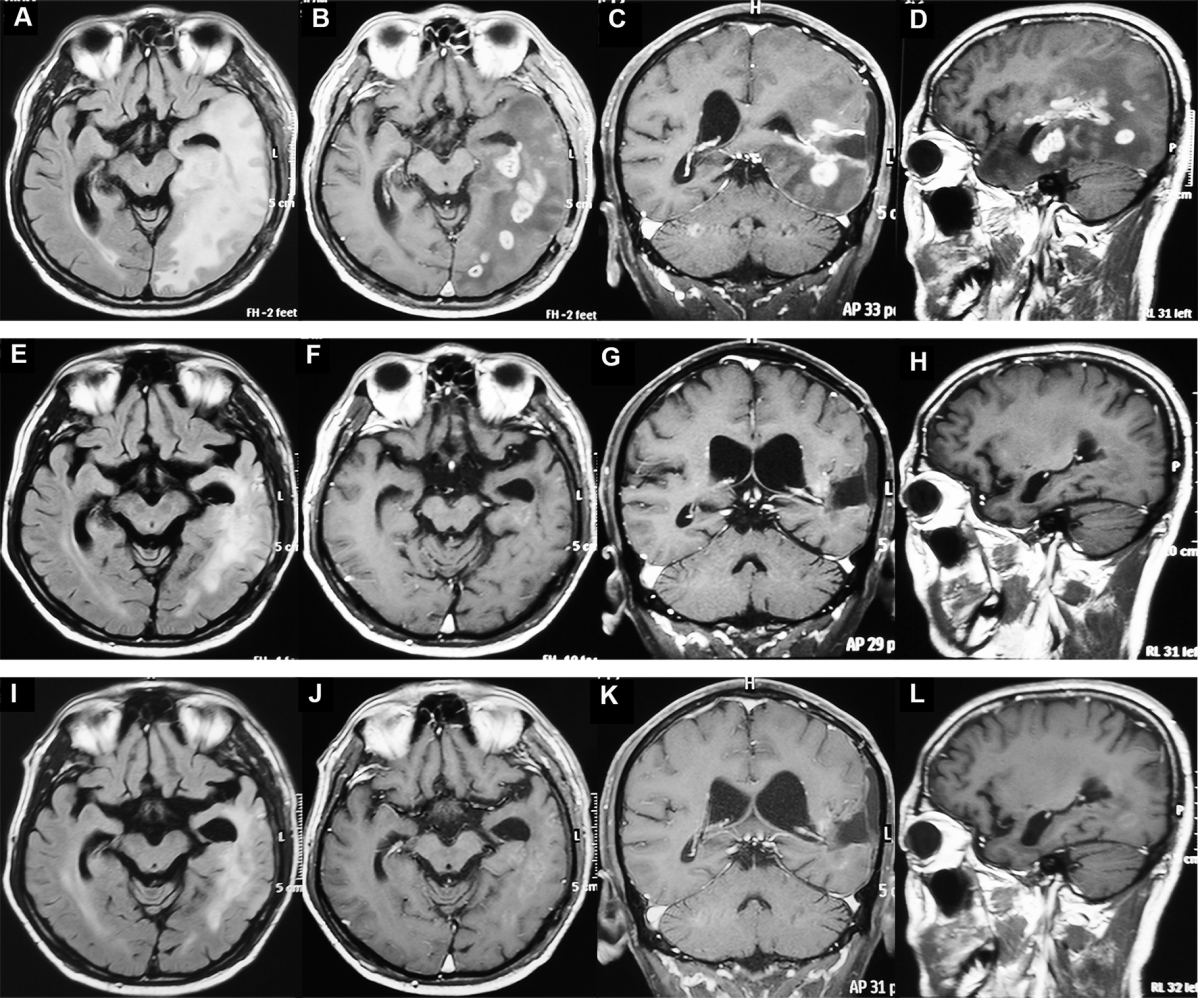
MRI of one patient with recurrent glioblastoma before and after apatinib plus temozolomide treatment. (A–D) The MRI before treatment. The tumors were enhanced and intracranial disseminated. (E–H) The MRI after two cycles of treatment. The enhanced tumors disappeared and the non-enhanced lesions (Flair) were decreased. (I–L) MRI after three cycles of treatment. The patient had a complete response.
